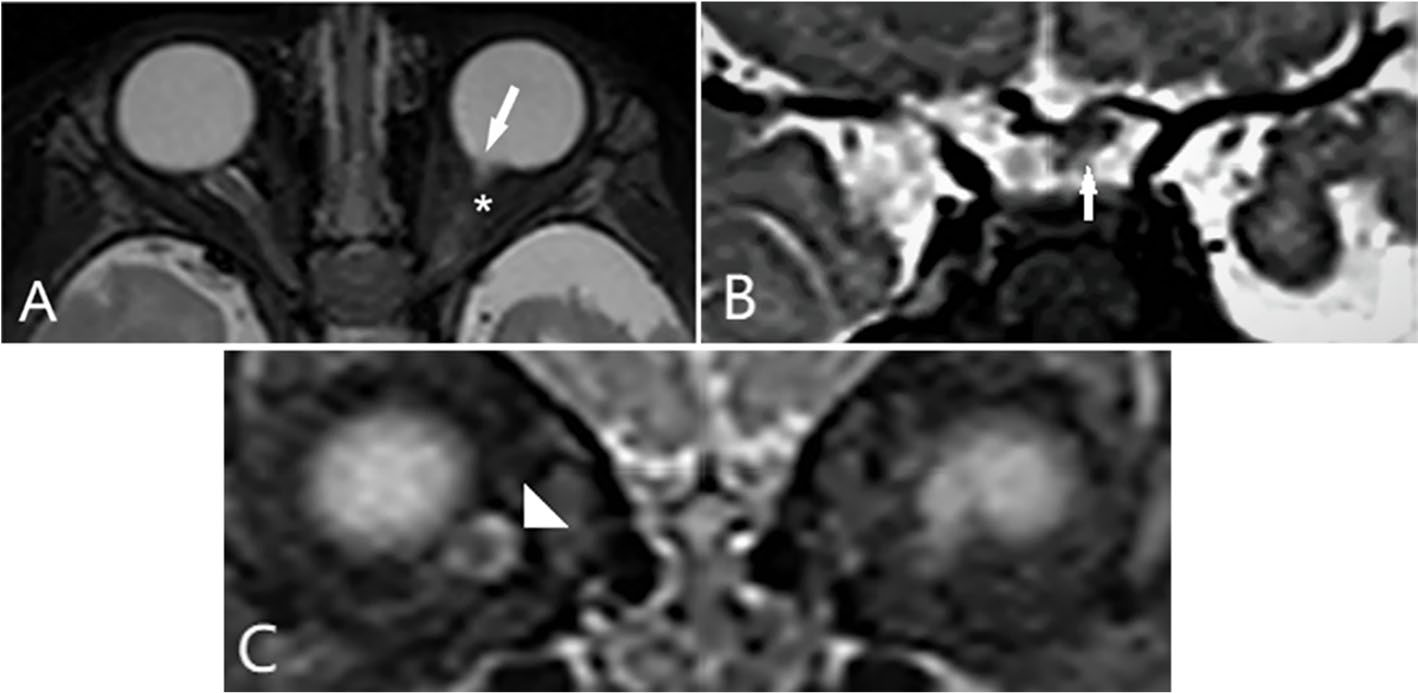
Title, MRI of the orbit. Legends, Axial T2WI through the orbits of the patient demonstrated (A) excavated optic nerve head with scleral defect (white arrow) and tortuous optic nerve (white star). The coronal T2W1 image (B and C) showing asymmetry of optic chiasma with distortion in left side (white arrow); right optic nerve surrounded by enhancing CSF (white arrow head) which is absent in left side
